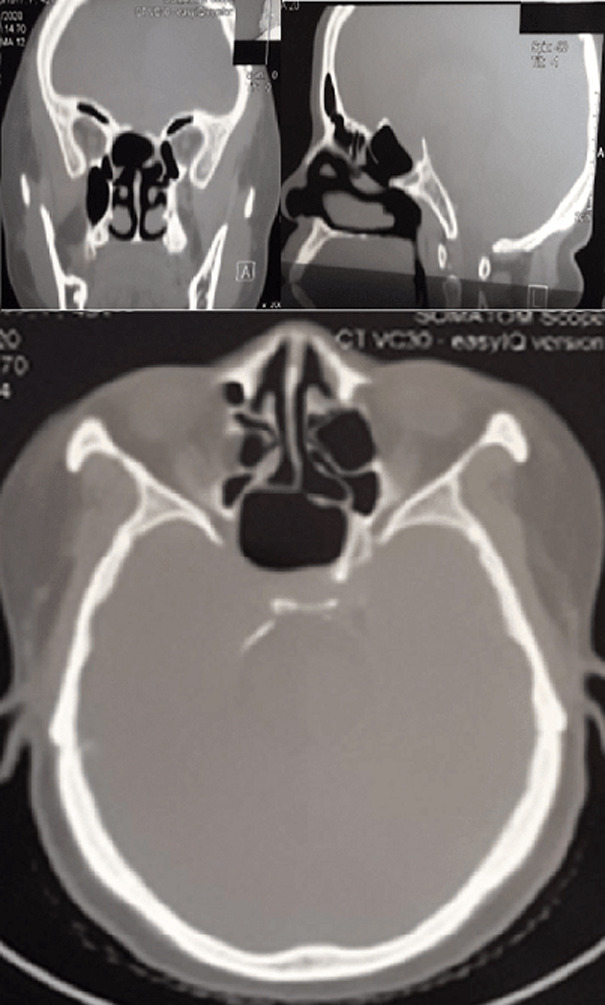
CT scan of the head, one month after the endoscopic surgical treatment, there is perfect aeration of the sphenoid sinus
